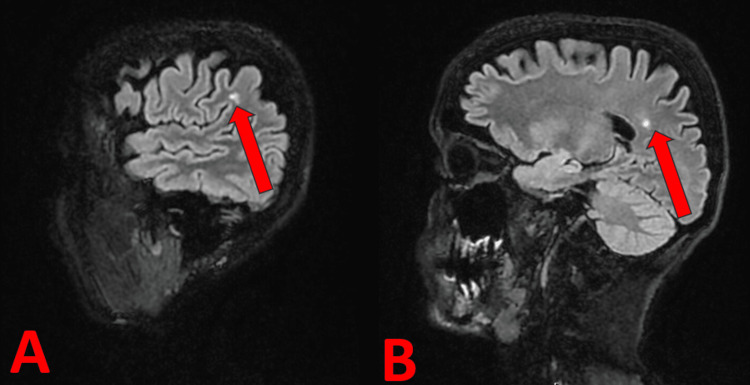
MRI (A) The MRI scan of the patient's brain (frontal section). The red arrow indicates small isolated hyperintense areas in the white matter. The scans come from the hospital records. (B) The MRI scan of the patient's brain (sagittal section). The red arrow indicates small isolated hyperintense areas in the white matter MRI: magnetic resonance imaging
